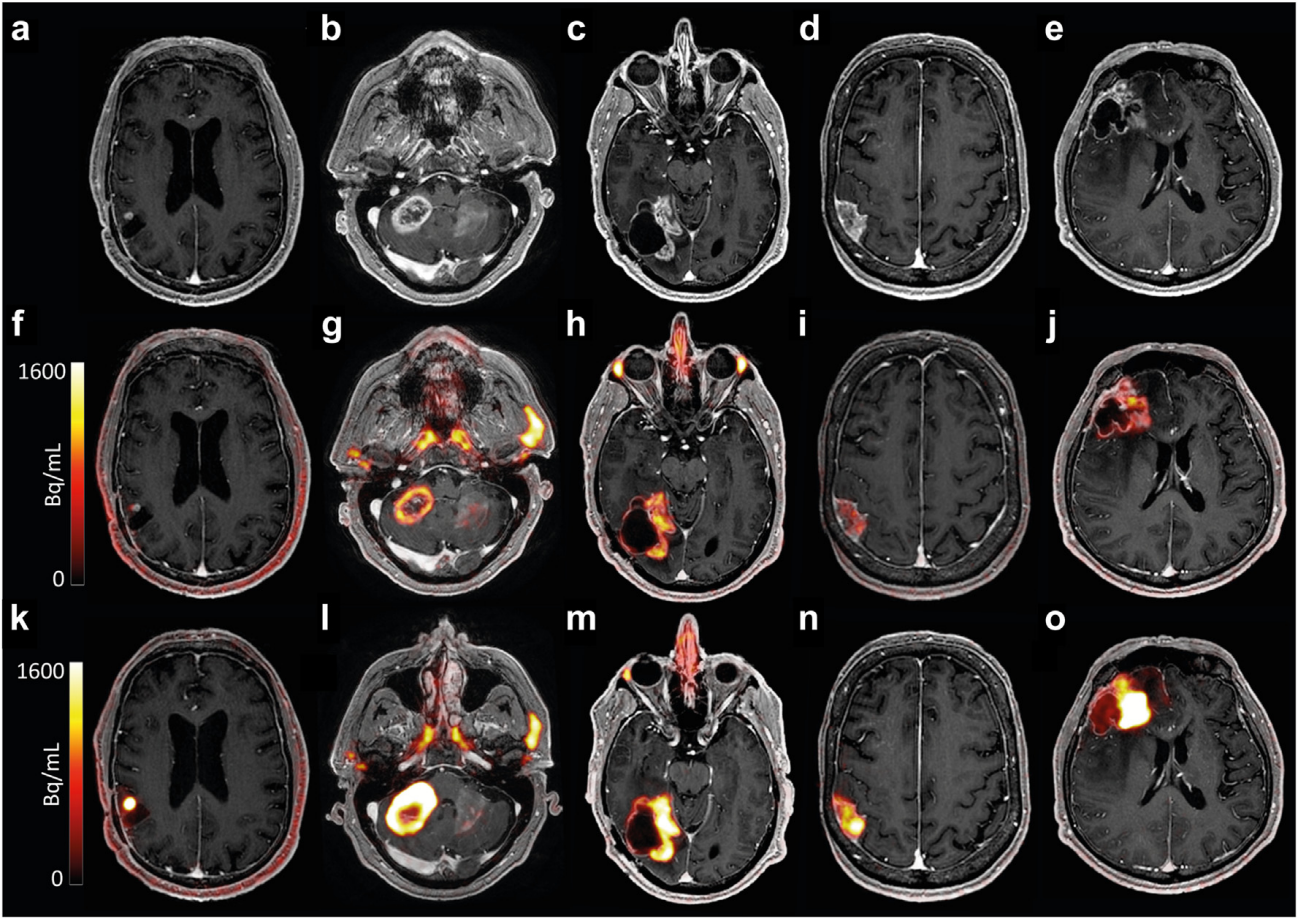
Uniformly scaled representative images of each patient with HGG (from left to right, no. 1–5) including a post-contrast T1w MR image (a–e), and fused [^68^Ga]Ga-PSMA-11 PET with post-contrast T1w MR images after IV (f–j) and ssIA administration (k–o). Abbreviations: HGG: high-grade glioma; IV: intravenous; PSMA: prostate-specific membrane antigen; ssIA: super-selective intra-arterial.
